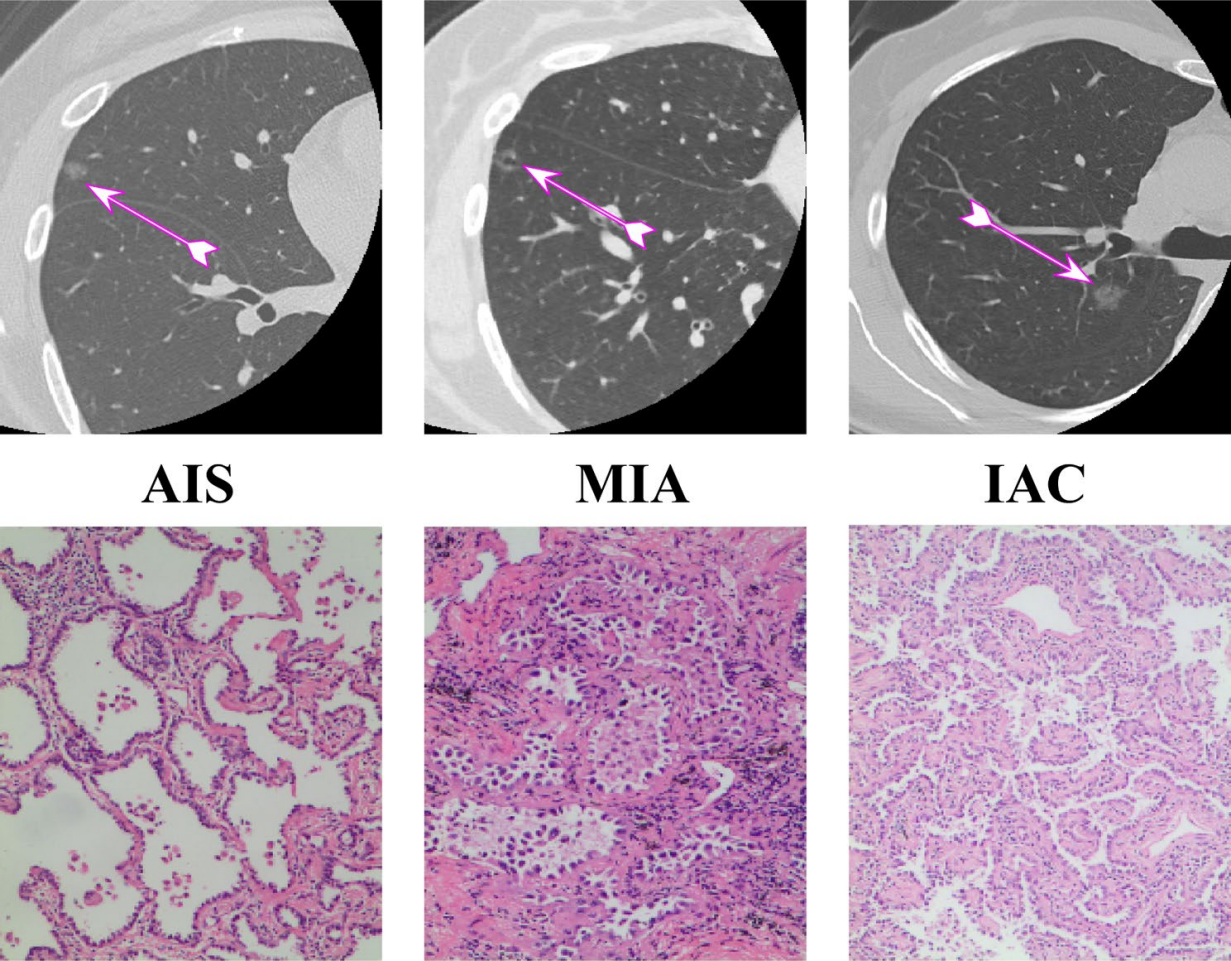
CT images showed three pure ground glass nodules (arrows), pathologically representing AIS, MIA and IAC, respectively. Among them, cystic airspace was seen on the MIA nodule, and lobulation on the IAC nodule
